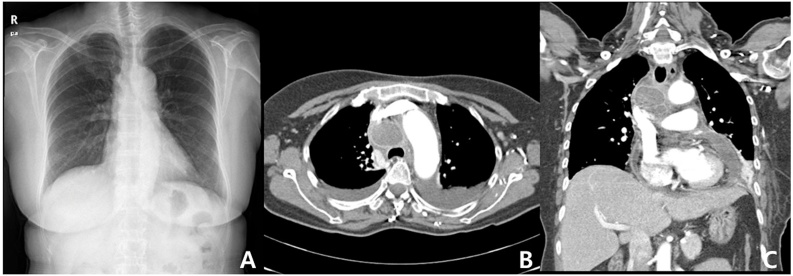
(A) Chest radiograph, performed 8 days after EBUS-TBNA, shows pleural effusion in both lungs, enlarged heart margin, and the size of the shadow previously observed in right hilar region increased further; (B, C) chest CT, performed 8 days after EBUS-TBNA, shows large amount of pericardial effusion, enlargement of the right lower paratracheal lymph nodes, and a multiloculated mediastinal abscess with gas formation along with the appearance of pleural effusion in both lungs.
